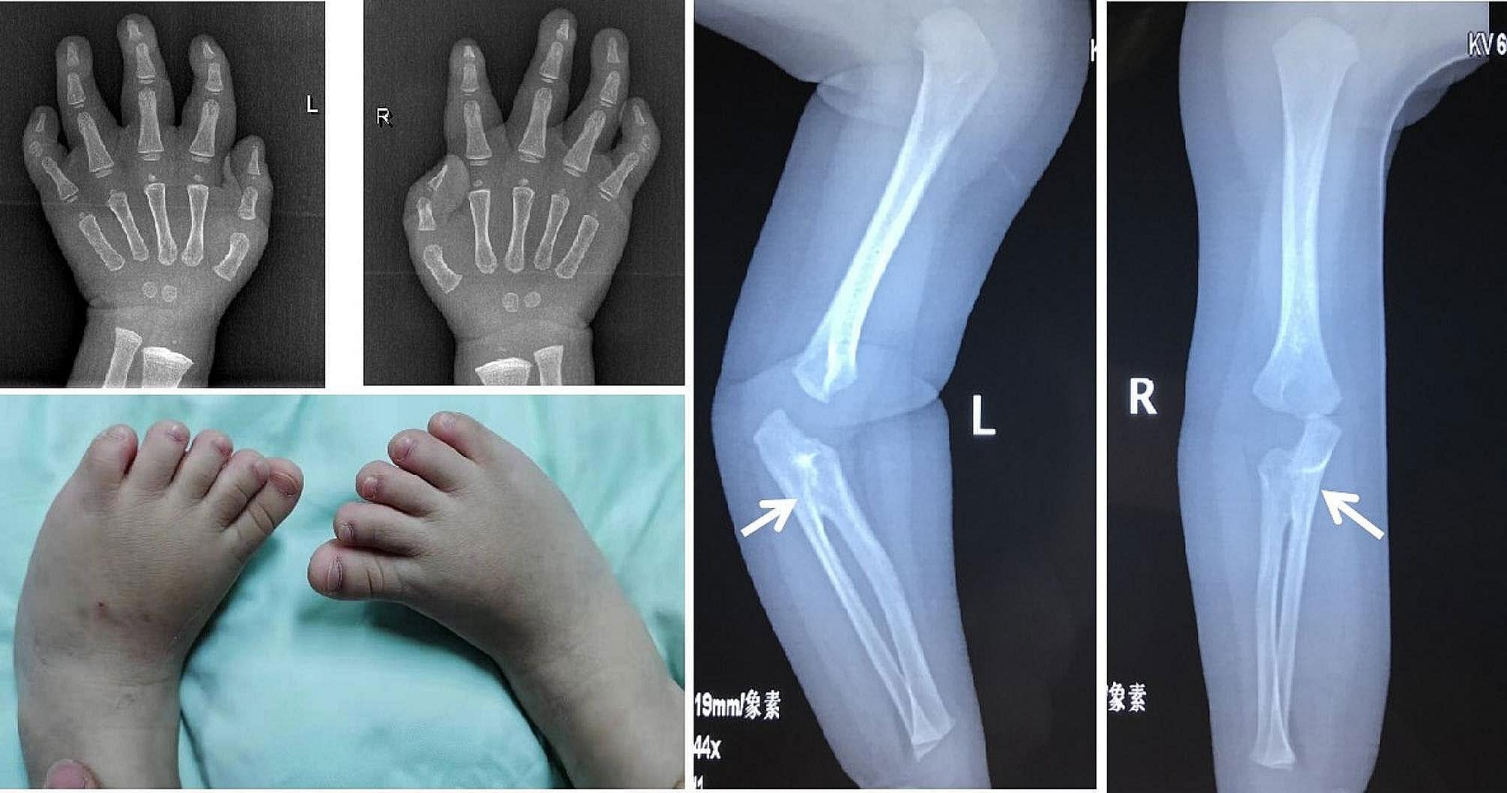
Radiographs of the patient show bilateral radioulnar synostosis (white arrows). Bilateral hands and feet were clinodactyly of digits
